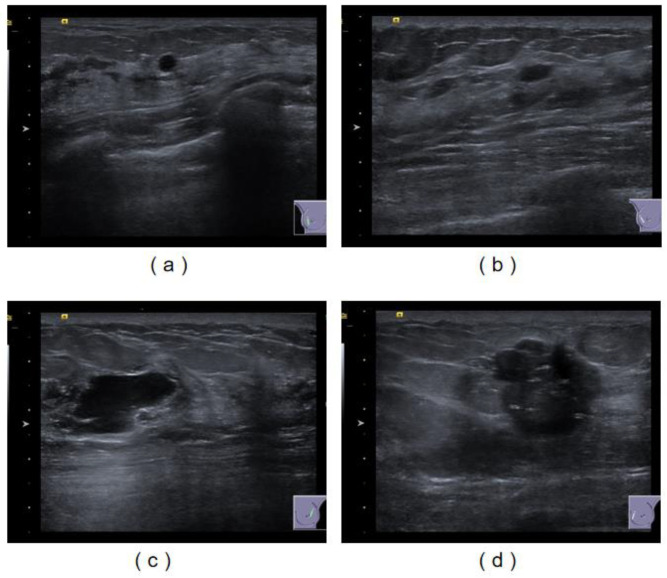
Ultrasound image of breast tumor. Among them, (a,b) are benign tumors, while (c,d) are malignant tumors.
